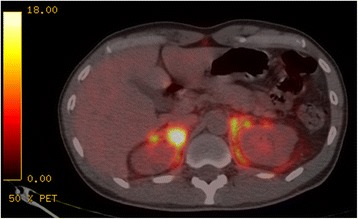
The iodine-123-metaiodobenzylguanidine (MIBG) scintigraphy image. MIBG scintigraphy showed increased uptake of MIBG in both adrenal glands, which was consistent with the diagnosis of bilateral pheochromocytomas
